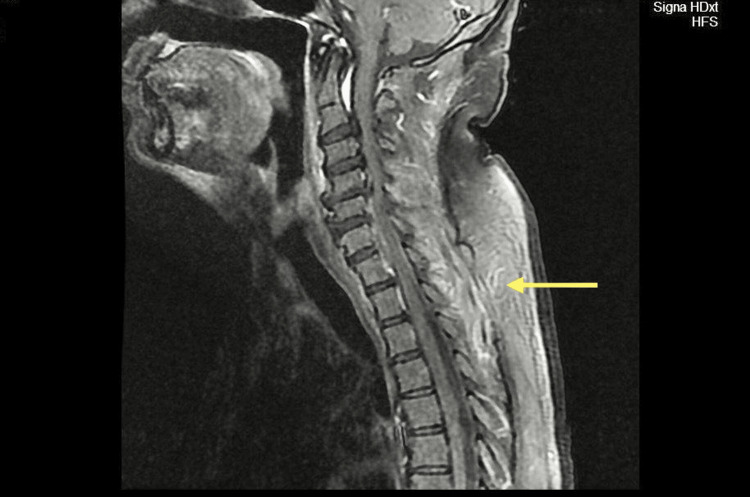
MRI of the cervical spine in sagittal view showing a CEA, as indicted by the yellow arrow. CEA: Cervical epidural abscess
